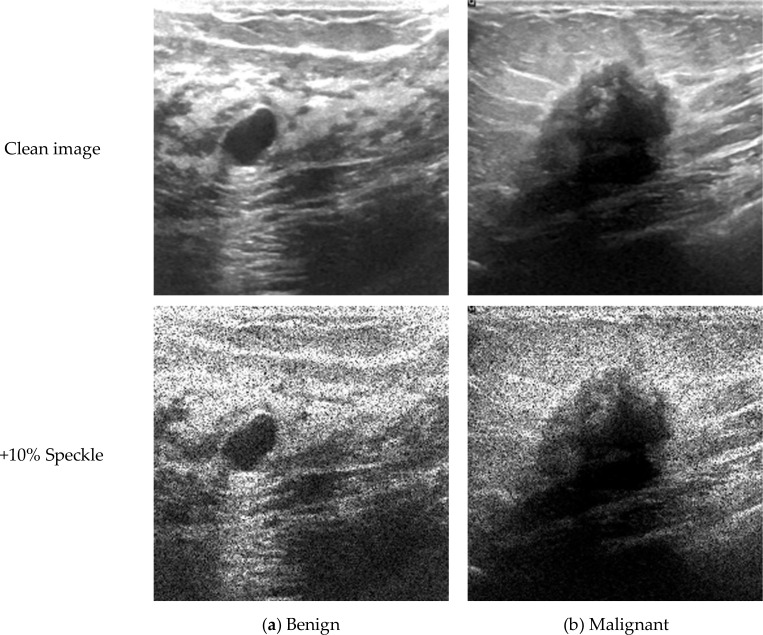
Representative examples from the Kaggle Ultrasound Breast Images dataset showing benign (left) and malignant (right) cases before (top row) and after (bottom row) applying 10% multiplicative Gaussian speckle noise (
